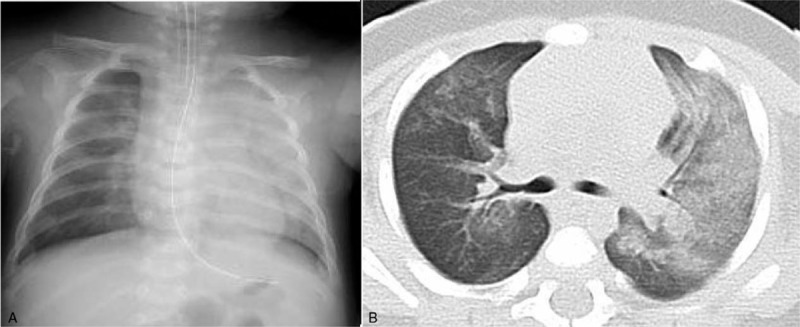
Case 1: (A) Chest radiograph showing diffuse ground glass opacification of the left lung; (B) Computed tomography image showing consolidation in the left upper and lower lobes.
